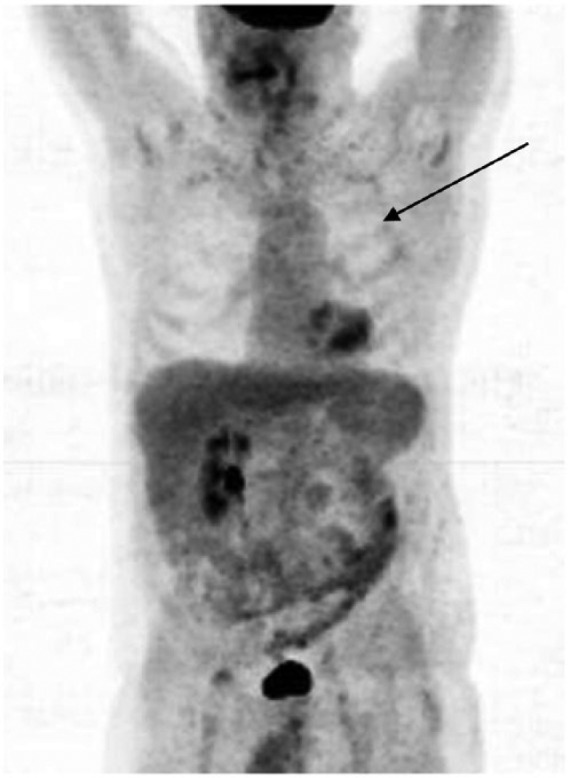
PET/CT scan, September 2013.
